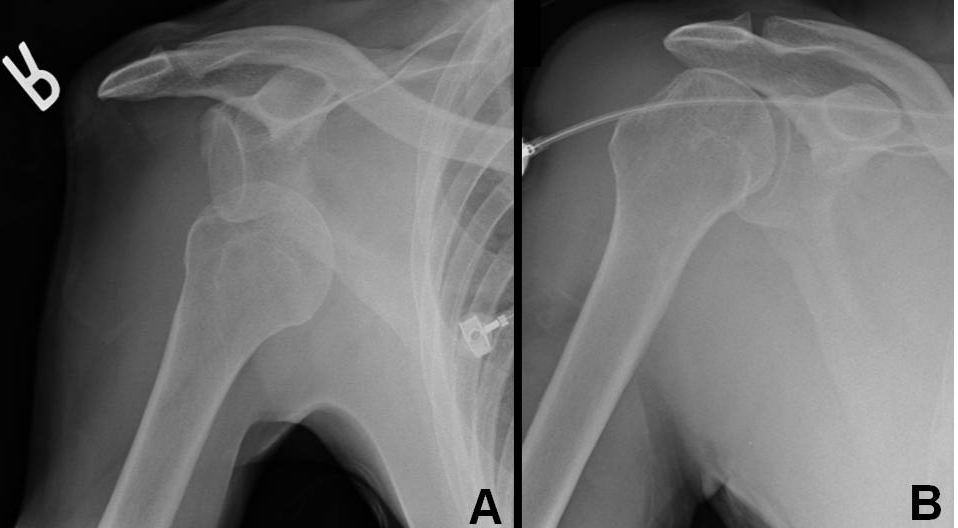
Case demonstration of a 46-year-old right hand dominant male who sustained a fall from standing onto the right shoulder. Injury X-ray demonstrates an anterior shoulder dislocation (panel A) which was successfully reduced in the emergency department (panel B).
